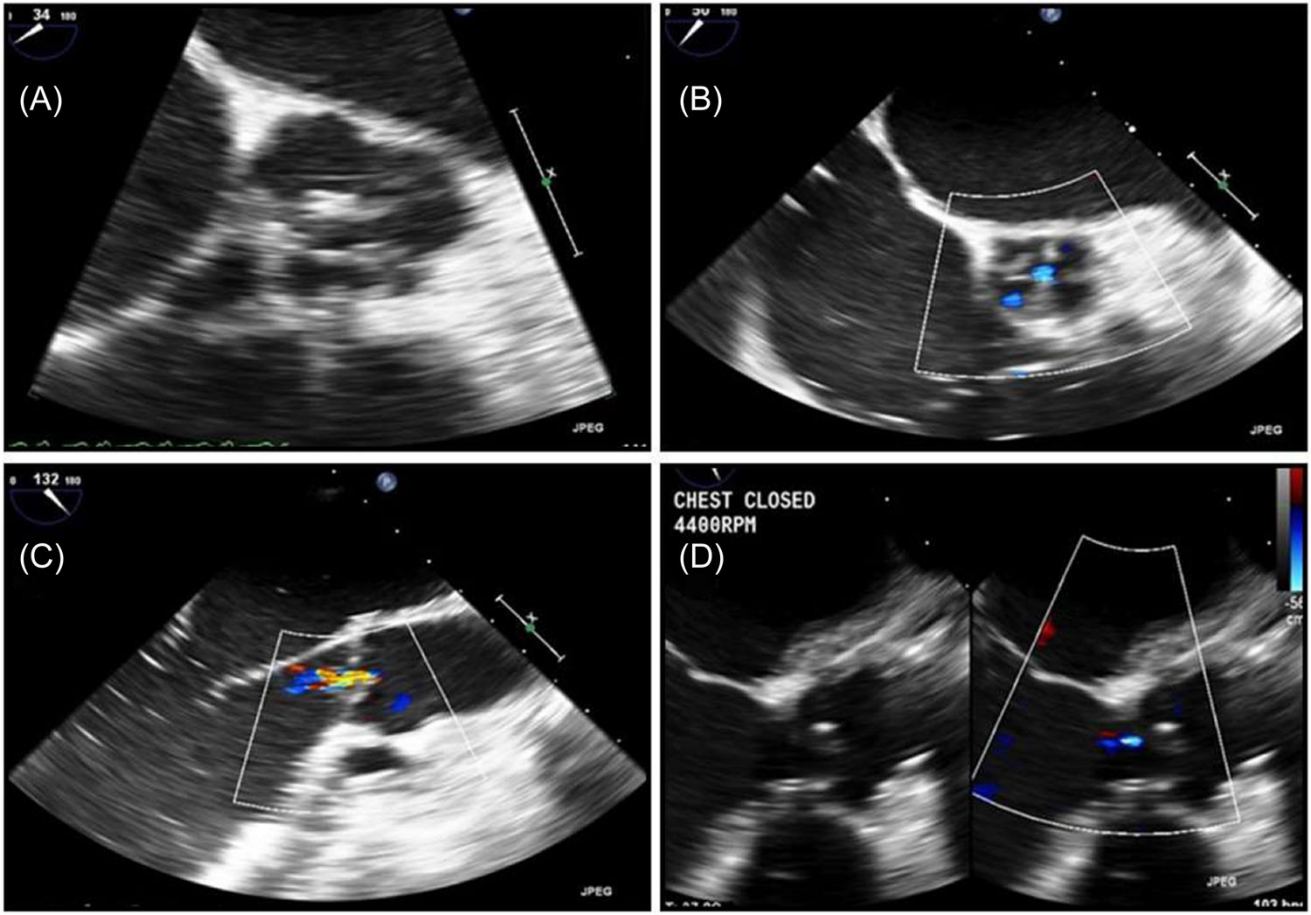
(A) Precardiopulmonary bypass, mid‐esophageal aortic valve short axis view with Impella in place crossing the aortic valve between the noncoronary and right coronary cusps. (B) On cardiopulmonary bypass following removal of Impella device, mid‐esophageal aortic valve short axis with color flow doppler demonstrating two AI Jets, one central and one at the location of the removed Impella. (C) On cardiopulmonary bypass following removal of Impella catheter, mid‐esophageal aortic valve long axis view demonstrating the central aortic insufficiency jet. (D) Postcardiopulmonary bypass and aortic valve repair, mid‐esophageal aortic valve long axis view demonstrating trace aortic insufficiency through a central jet
